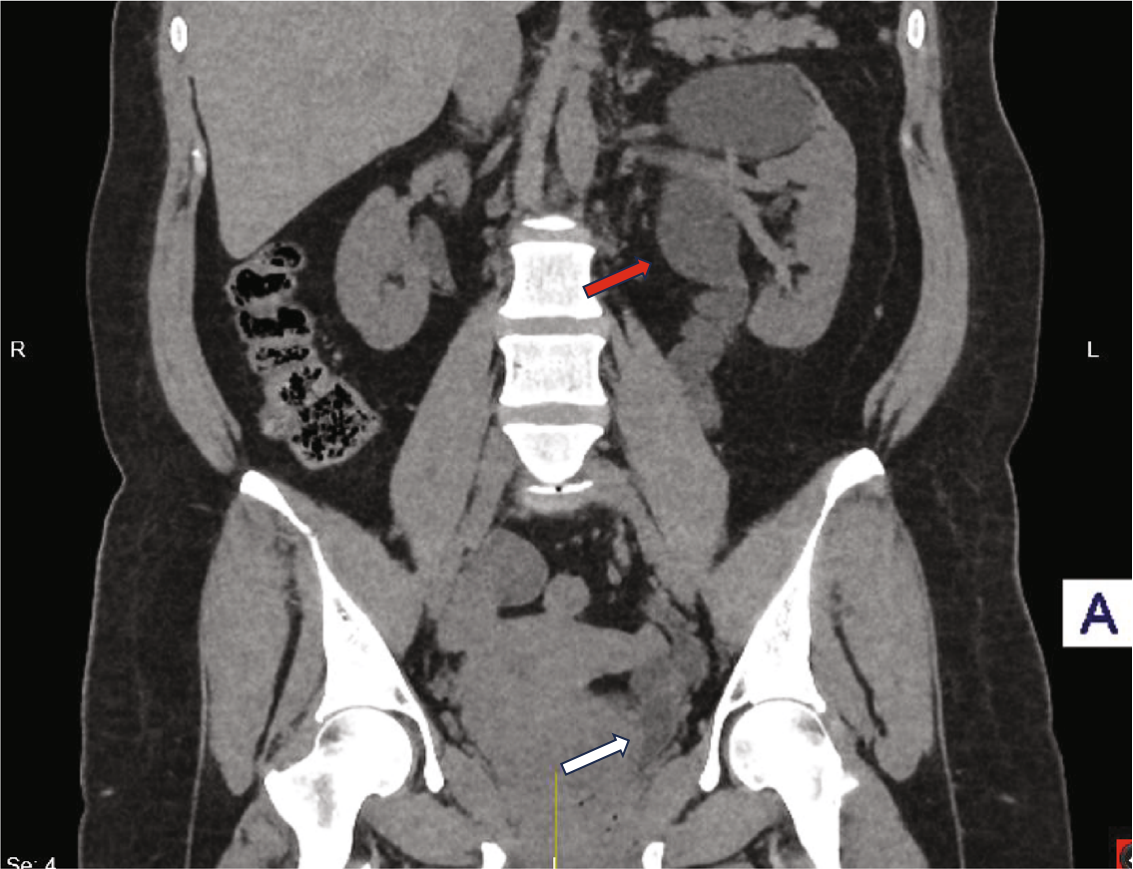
CT urinary tract, 2 months postoperatively: coronal section. Persistent dilated upper moiety (red arrow). No evidence of any remaining calculus (white arrow).
